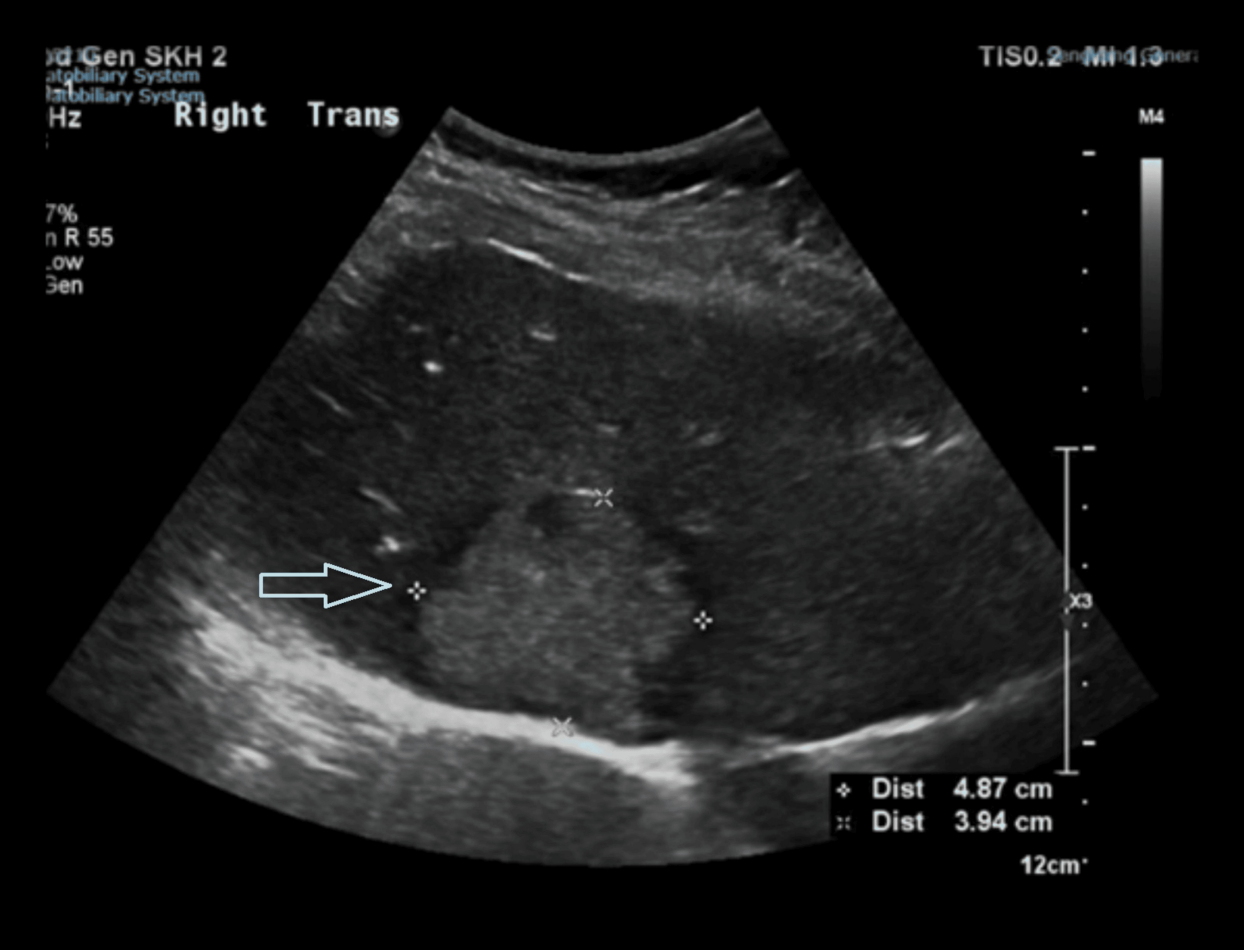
Ultrasound of the liver showing an echogenic lesion in the right hepatic lobe with peripheral hypoechoic halo measuring 4.5 x 4.9 x 3.9 cm
